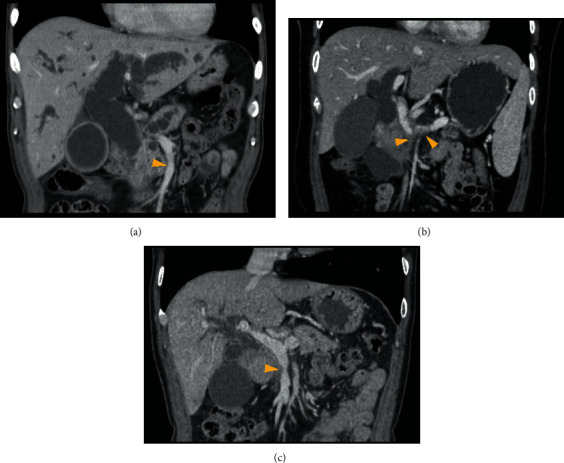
Preoperative computed tomography scans for Patients 1, 2, and 3 (a–c). (a) SMV invaded by a pancreatic tumor; (b) SMV and splenic vein invaded by pancreatic head tumor; (c) SMV invaded by pancreatic head tumor. Yellow arrowheads indicate areas of tumor invasion of blood vessels. SMV: superior mesenteric vein.
